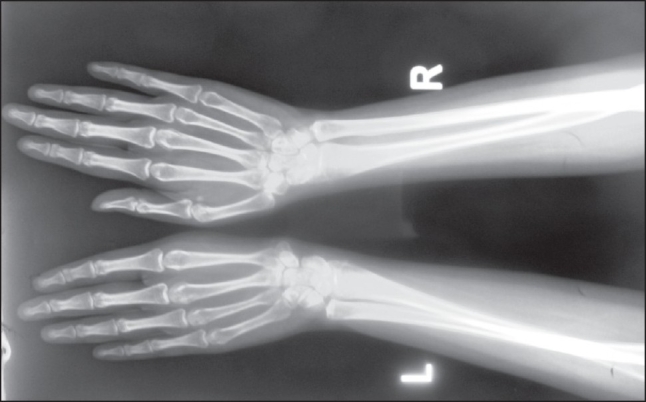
Plain radiograph of the hands.What are the abnormal findings on this abdominal CT scan (Figure 2)?
